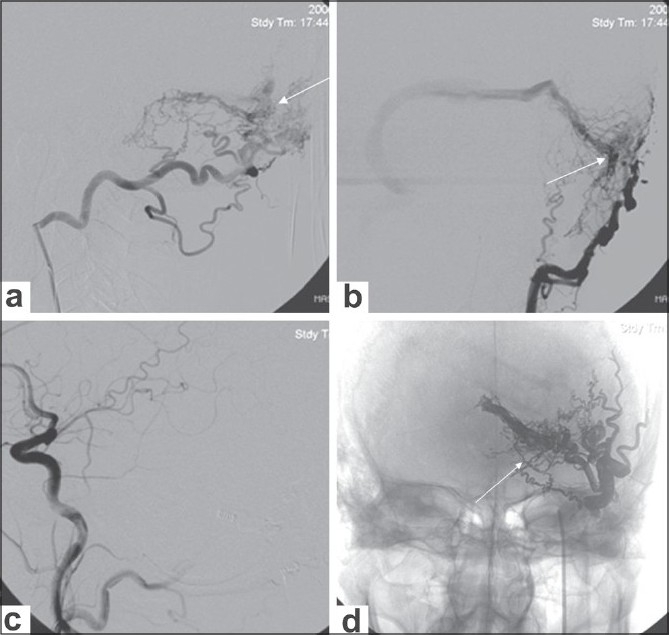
Left transverse dural arteriovenous fistula (AVF). Lateral (a) and oblique (b) left occipital angiograms show a left transverse dural AVF (arrow). Transarterial embolisation was performed through the occipital artery with ONYX. Postembolization left common carotid angiogram (c) shows occlusion of the fistula. Plain radiograph (d) shows the ONYX cast (arrows)
